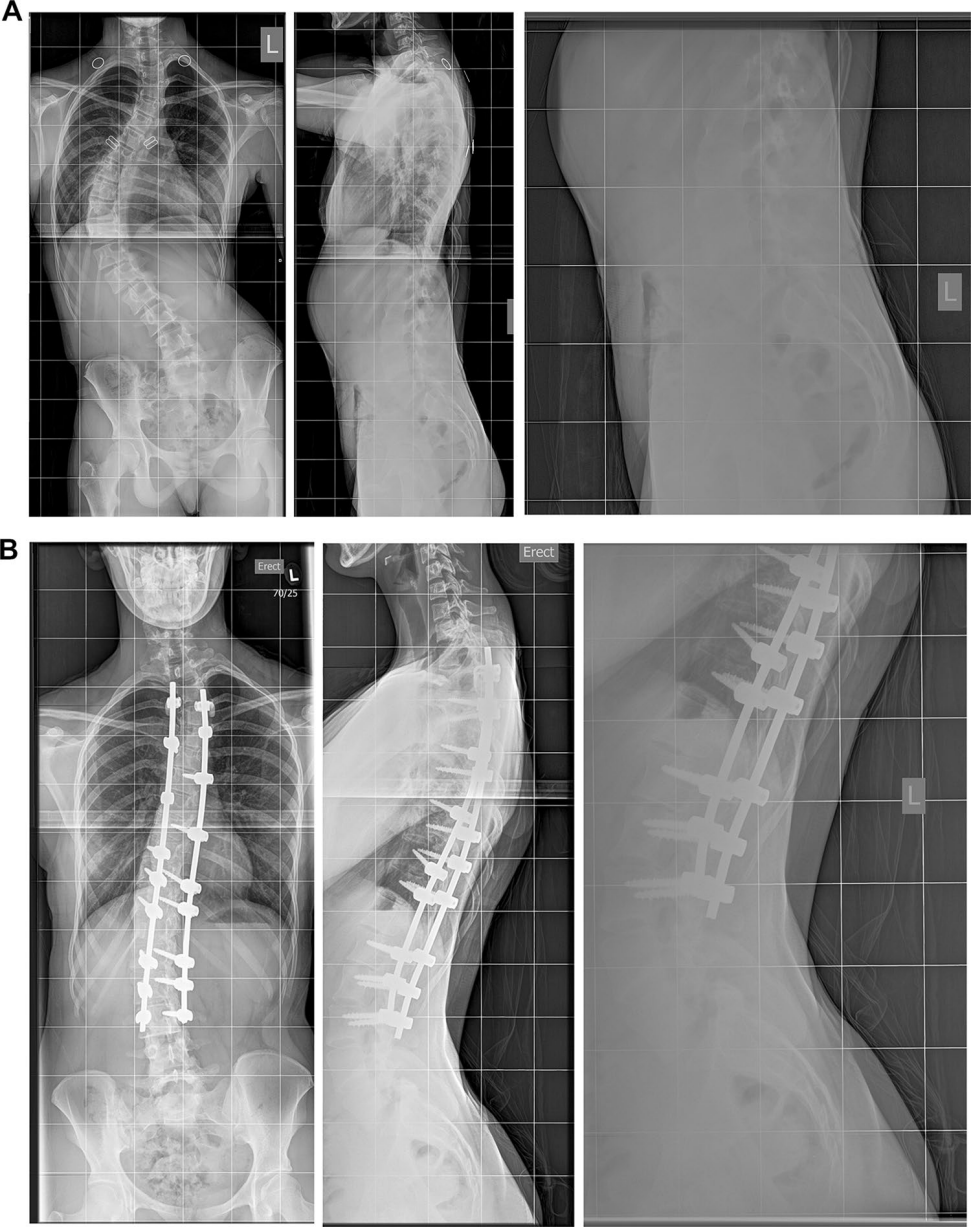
Clinical example of a 13-year-old girl with a 70° curve and a grade 1 isthmic spondylolisthesis (A). Four years after T3-L3 posterior fusion (LIV = EV), the X-ray showed a stable correction, there was no progression of the slippage and the patient was pain free (B)
